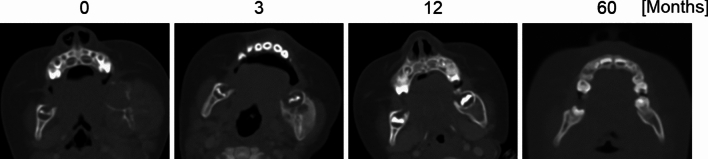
Representative CT images showing the transition from bone destruction to remineralization and bone remodeling in a 9-year-old patient. Bone destruction is observed in the left mandible at 0 months. Excessive remineralization was observed at 3 months, followed by bone remodeling at 12 months, resulting in a morphology approximating the anticipated normal contour at 60 months
